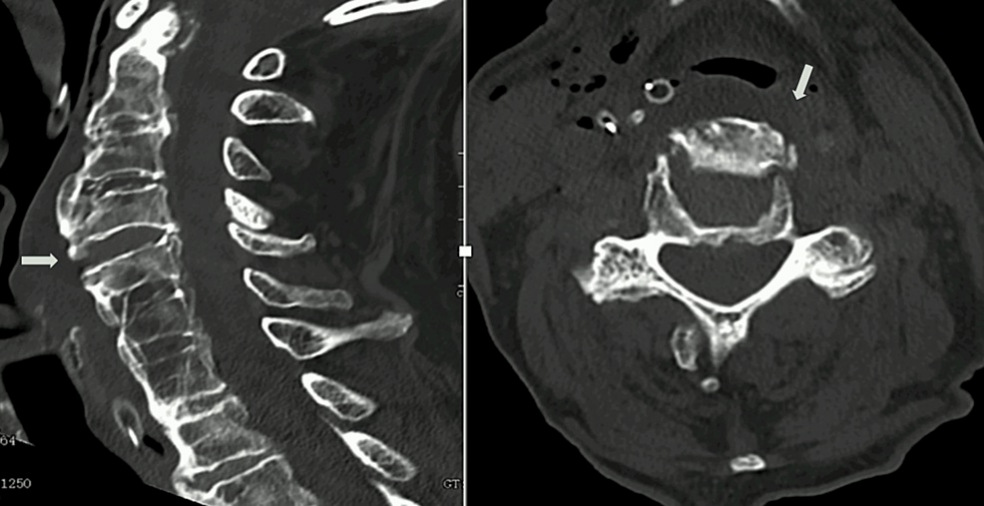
Postoperative cervical CT scan revealing adequate removal of cervical osteophytes responsible for extrinsic esophageal compression.
